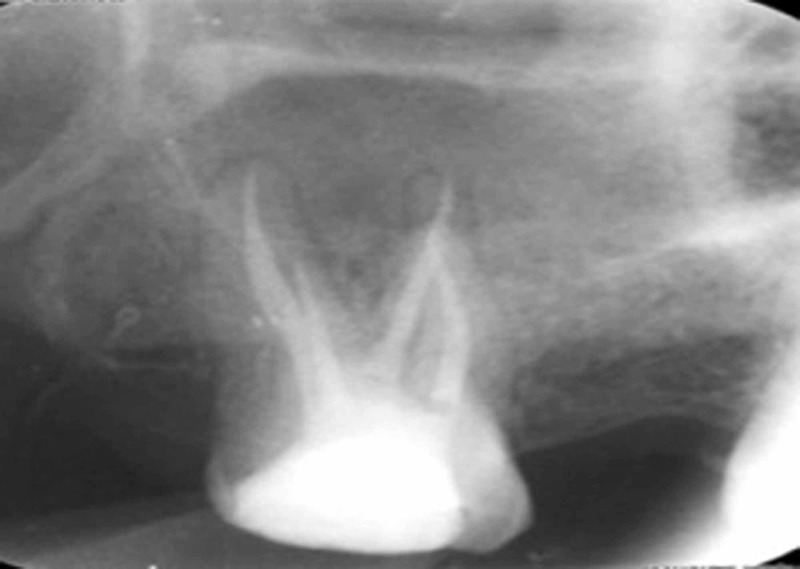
Follow-up radiograph of #27 after six months
